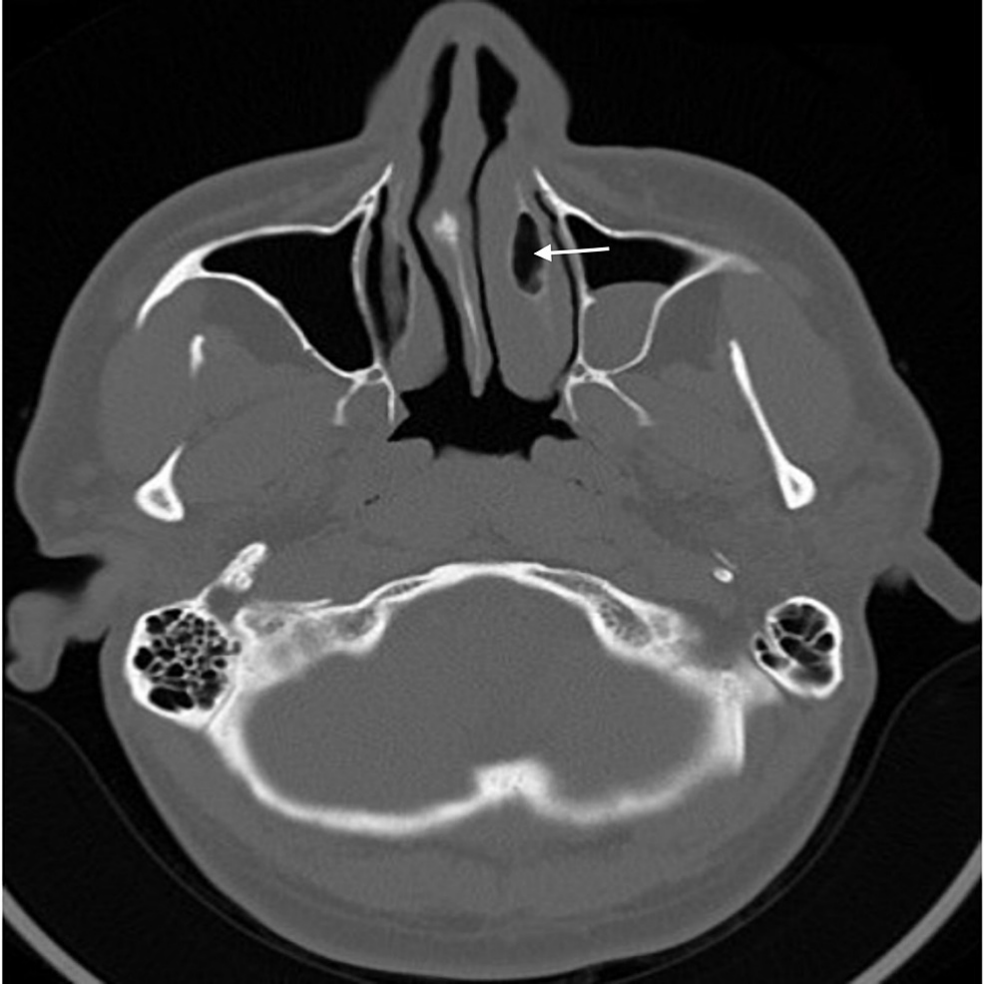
CT image of patient 1 (axial view) Axial view demonstrates CB of the left inferior turbinate (white arrow) CB: Concha Bullosa
